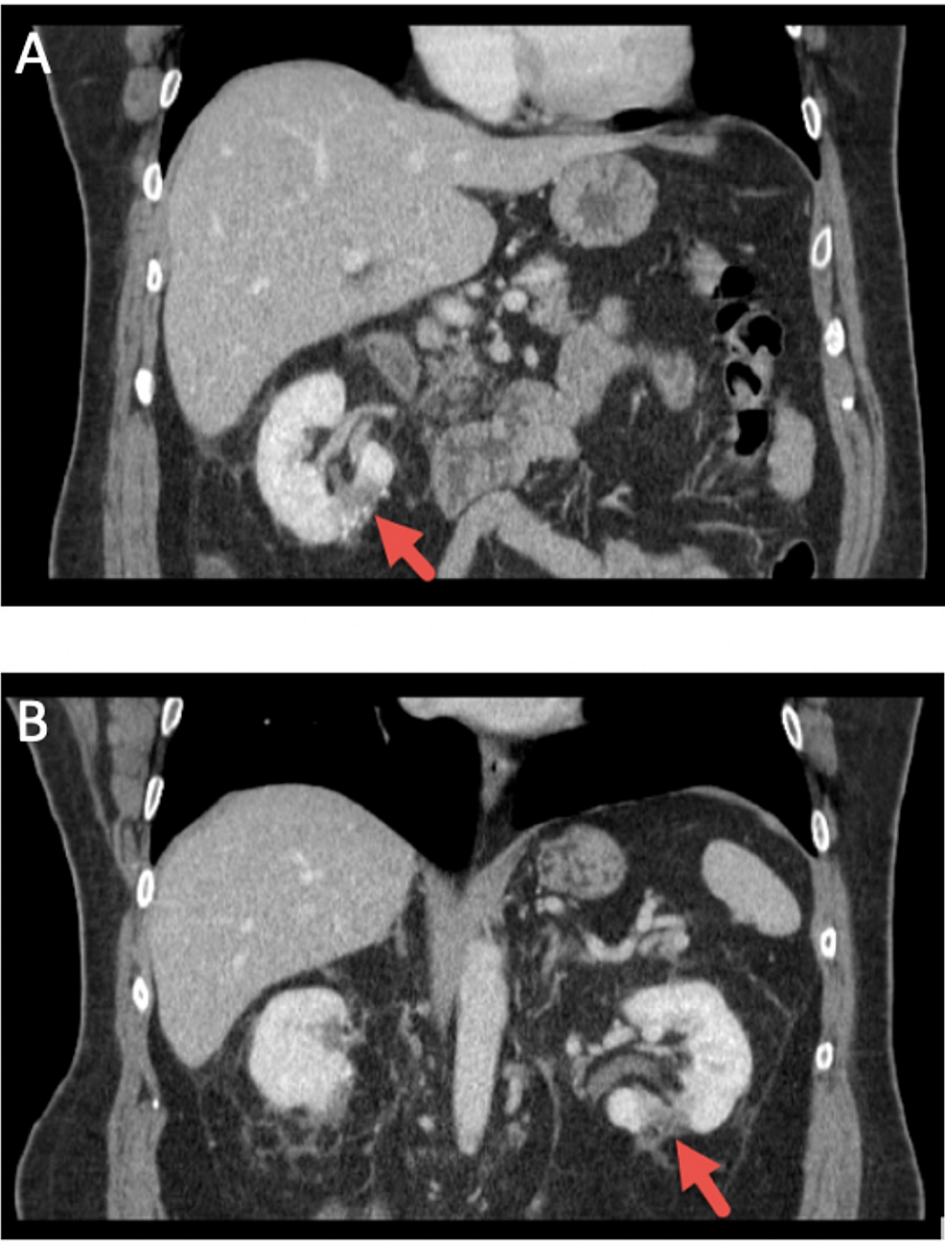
CT abdomen pelvis of a 66-year-old man with bilateral renal oncocytoma. (A) Arrow points toward a 3.0 cm mass in the lower pole of the right kidney on CT. (B) Arrow points toward a 3.3 cm isodense mass on the left lower kidney on CT. CT, computed tomography.
